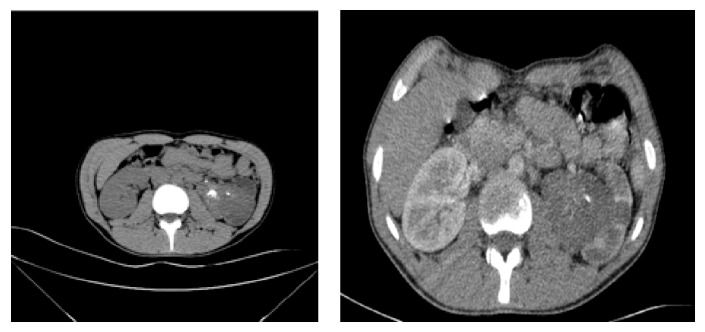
Preoperative CT with and without contrast.
